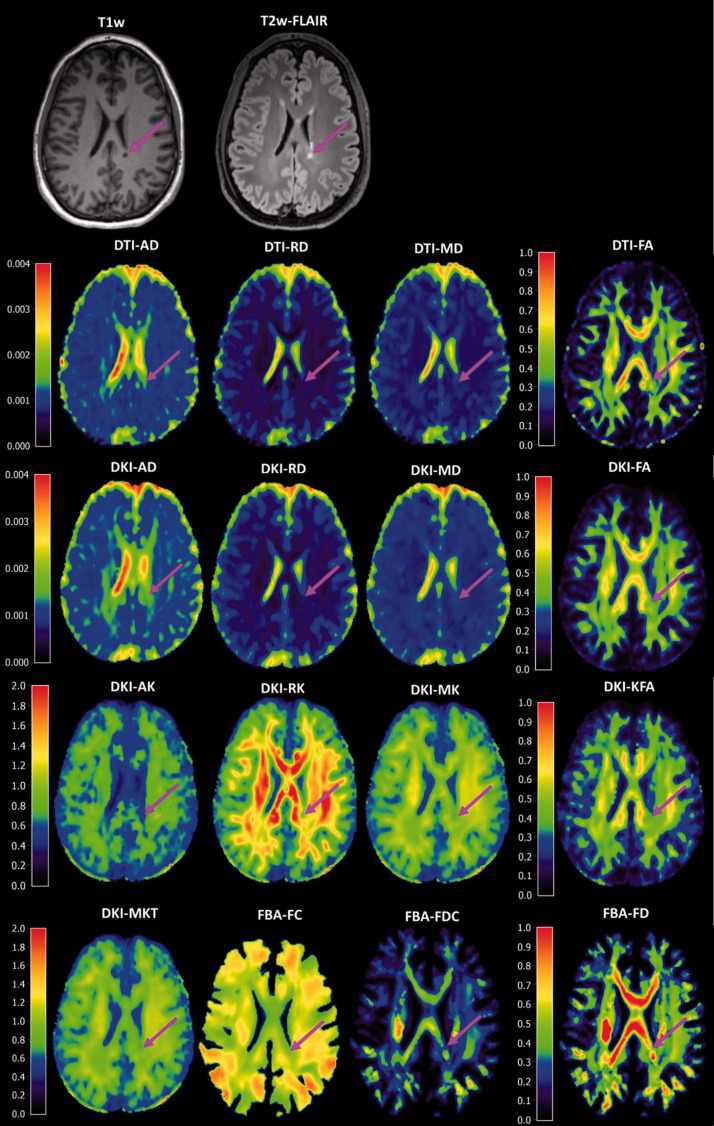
Structural T_1_w and T_2_w MRI with various diffusion MRI derived parameters. The brain figures are from one subject, with a black hole (pink arrow) on the presented slice. Close-up images of the lesion sites are provided in Appendix Figure 3.
